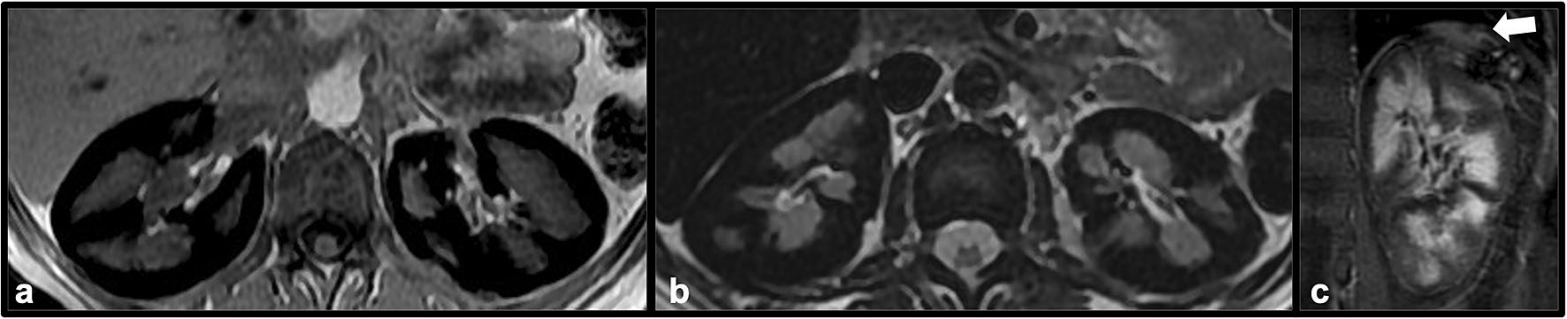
Axial GRE (a) and T2 (b) MR images in a patient with sickle cell disease demonstrate diffusely low renal cortical signal bilateral consistent with siderosis. The liver also demonstrates diffusely low T2 signal. Coronal post-contrast T1 image (c) demonstrates a small, largely infarcted spleen (white arrow)
